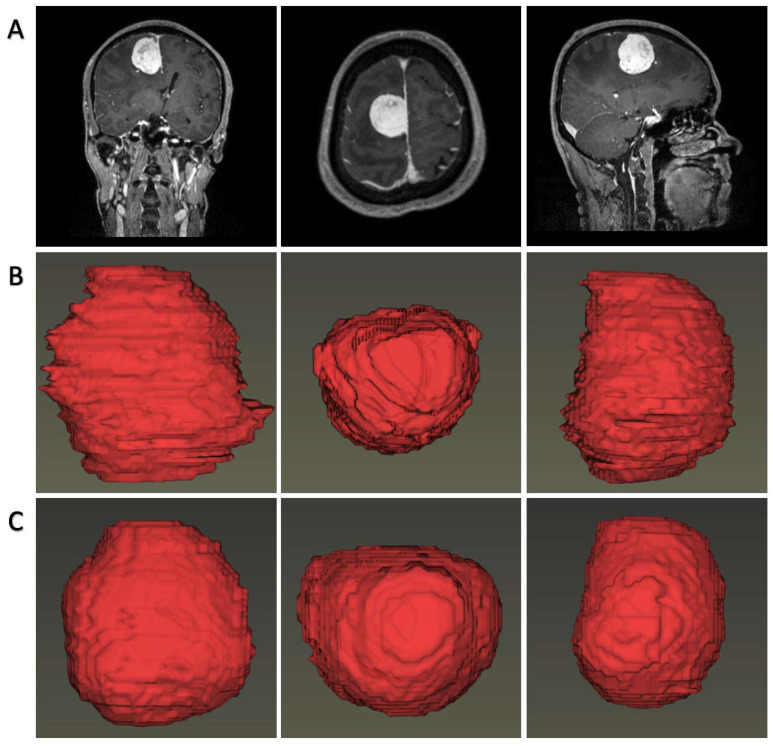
Visualized tumor reconstructions by automatic predicted labels and human-noted labels. (A). original MR images; (B). tumor rendering with human-noted labels; (C). tumors rendering with automatic predicted labels.
